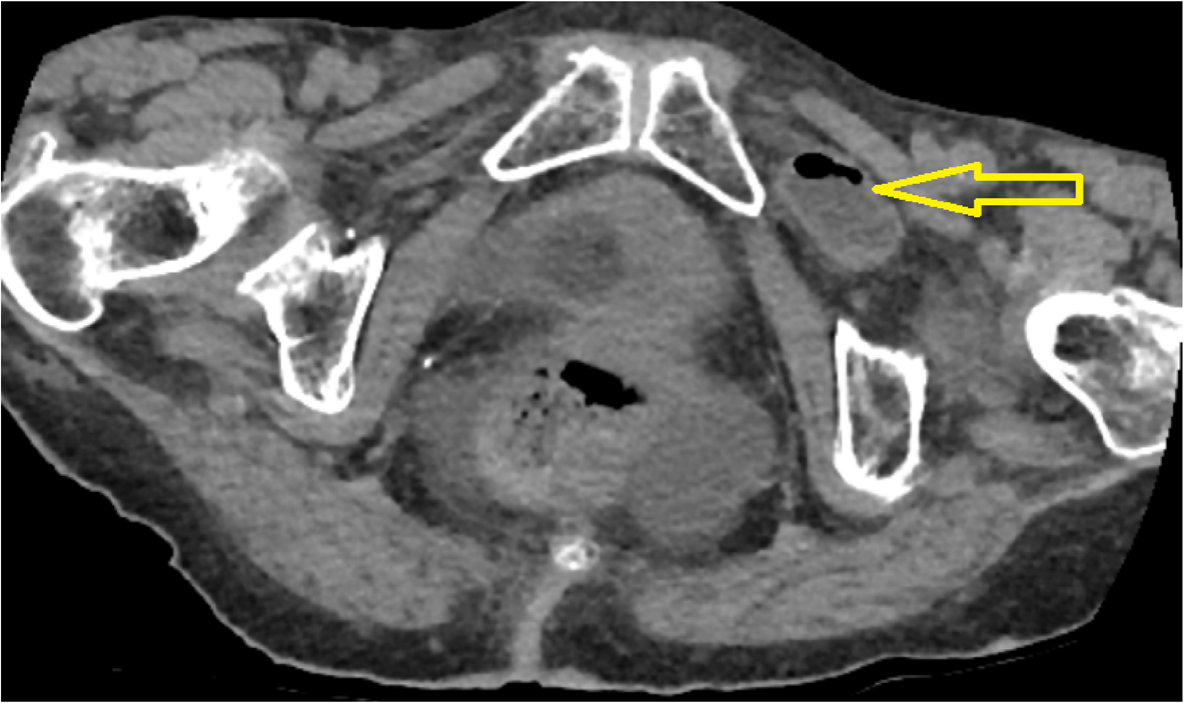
Transversal CT scan. The yellow arrow indicates bowel in the obturator foramen
